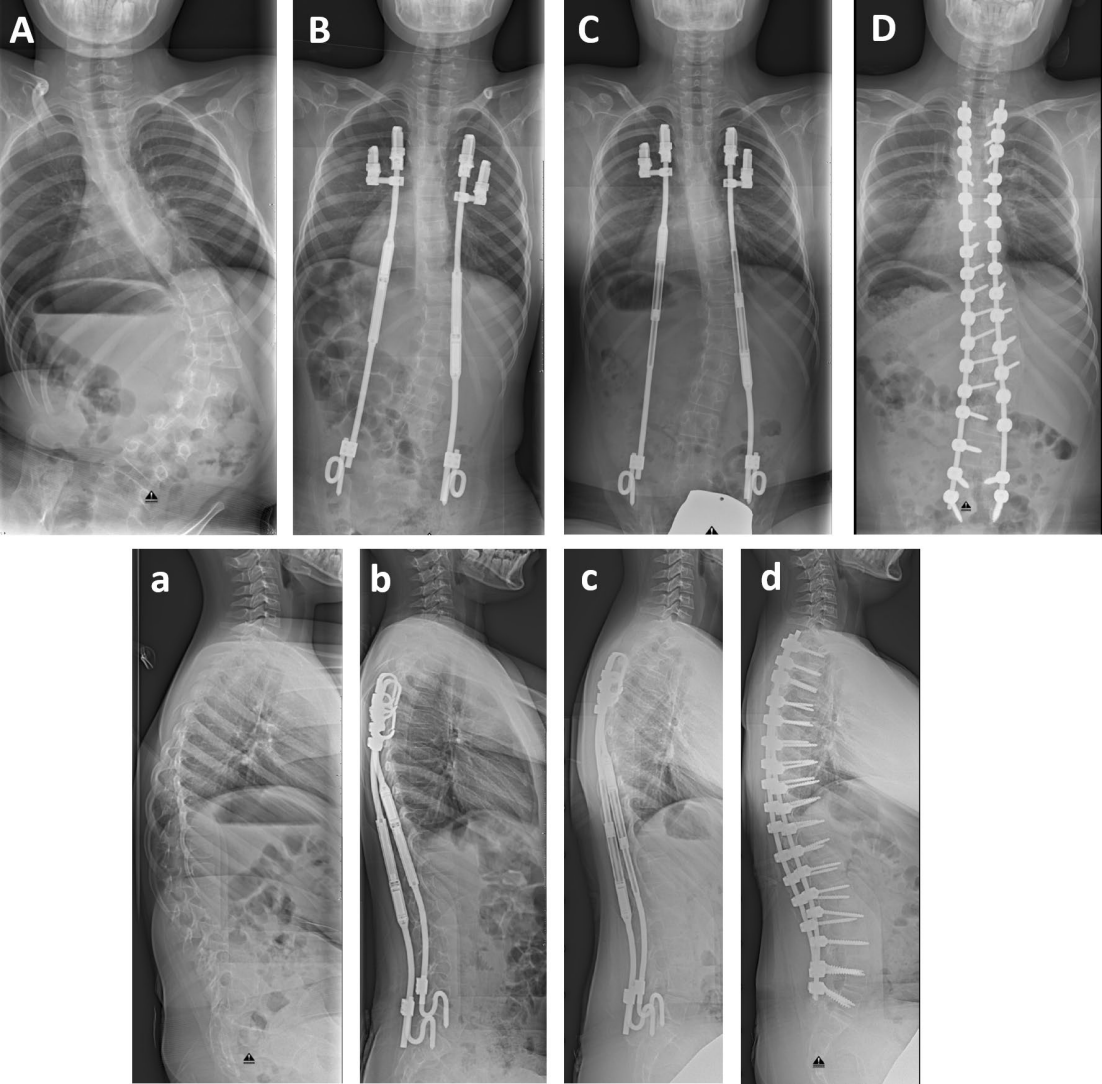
Posteroanterior (A–D) and lateral radiographs (a–d) of a nine-year old boy with SMA and spinal deformity. The scoliotic curve was corrected from 81° (A) to 27° (B) directly after MCGR implantation. This result could be maintained within the four-year follow-up (C) and after spondylodesis (D). The sagittal profile could be improved. Initial kyphosis of 42° (a) could be corrected to 29° directly after surgery (b) and to 36° during follow-up (c) and spondylodesis (35°, d).
